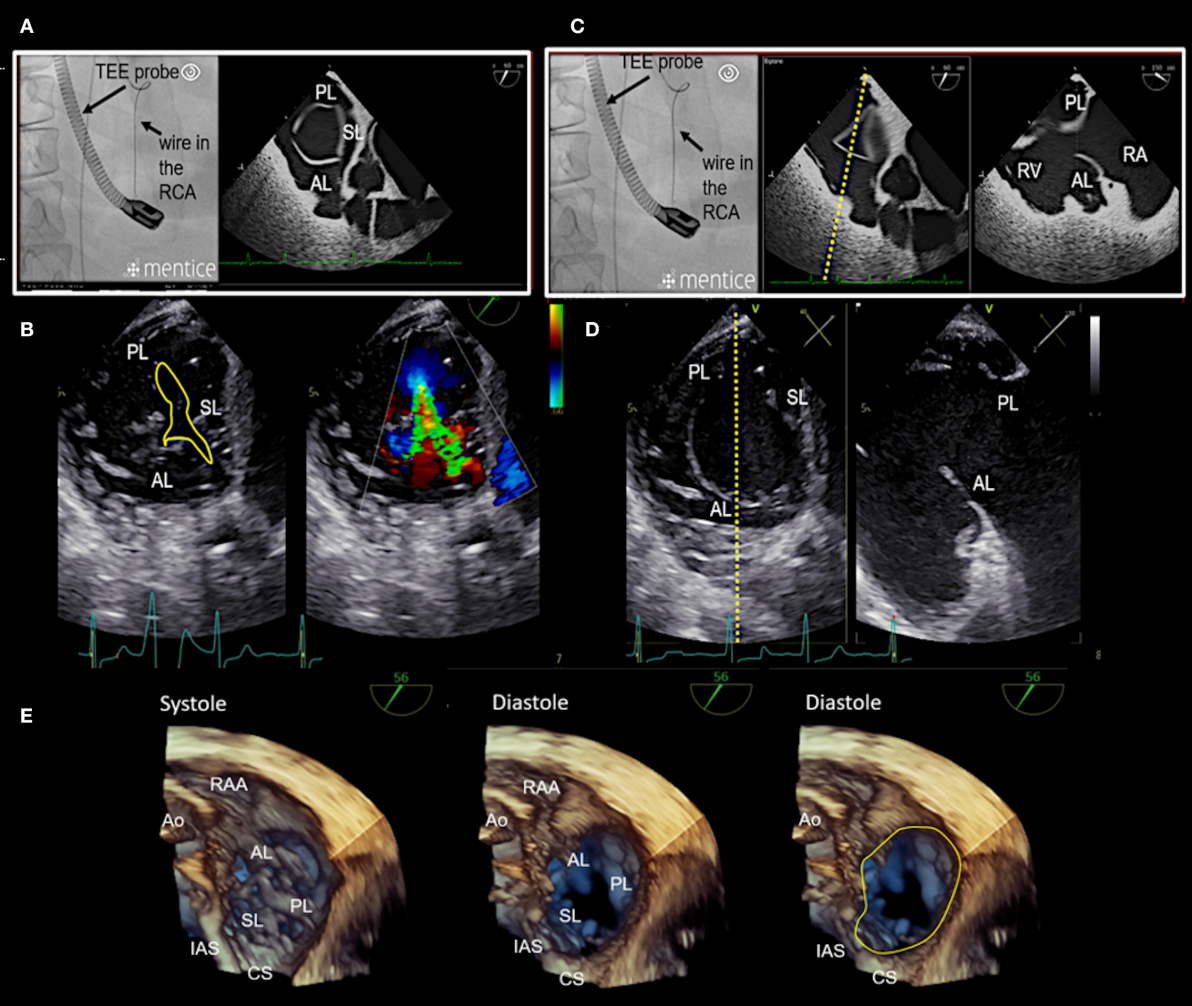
Transgastric and 3D enface views. Trans-gastric short axis views are particularly helpful, as all leaflets are usually visible. In most cases, adequate short axis views can be generated between 30 and 60°, so that the probe can be easily advanced trans-gastrally from the mid-esophageal RV inflow-outflow tract view. Additively an anterior flexion is then necessary. It should be attempted to display all commissures. (A) The image on the left shows the necessary positioning of the TEE probe in fluoroscopy (35° RAO, 6° CRANIAL) to create this trans-gastric short axis view at 60°. (B) A zoomed 2D TEE trans-gastric view obtained at 60° is shown. On the left the coaptation gap is traced (yellow line), on the right color Doppler is used to assess the origination and extension of the TR jet. (C,D) X-plane imaging along the anterior leaflet (images on the left) allows the evaluation of the anterior and posterior leaflet in relation to each other in long axis views (images on the right). (E) A three-dimensional (3D) enface view of the TV is acquired from a 60 degree view. It should be ensured, that part of the aortic valve/aortic root (Ao) and the coronary sinus (CS) is included in the 3D TEE data set. The image is than tilted and rotated so that the aorta is positioned at ~11 O‘clock and the CS at ~6 O‘clock. The entire circumference should be visible. The image on the left shows a 3D enface view in systole, in the middle a diastolic view is seen and on the right the delineation of the hinge-line is marked (yellow line) in the same view. Note the oval shape of the annulus in this case. RAA, right atrial appendage; IAS, interatrial septum; SL, septal leaflet; AL, anterior leaflet; PL, posterior leaflet; TEE, transesophageal echocardiography. Other abbreviations see Figure 3.
